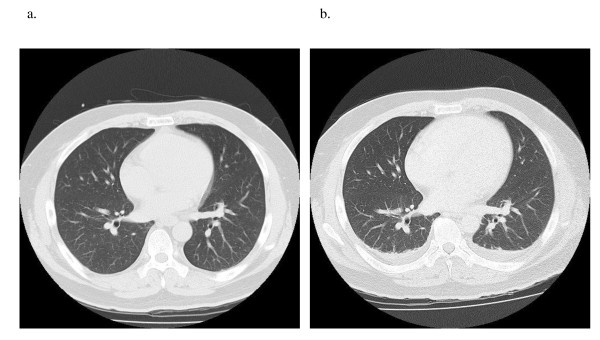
(a) Computed tomography of the chest. Hospital day 0. No abnormal findings. (b) Hospital day 2. Auxocardia and hydrothorax extending to both lungs.
